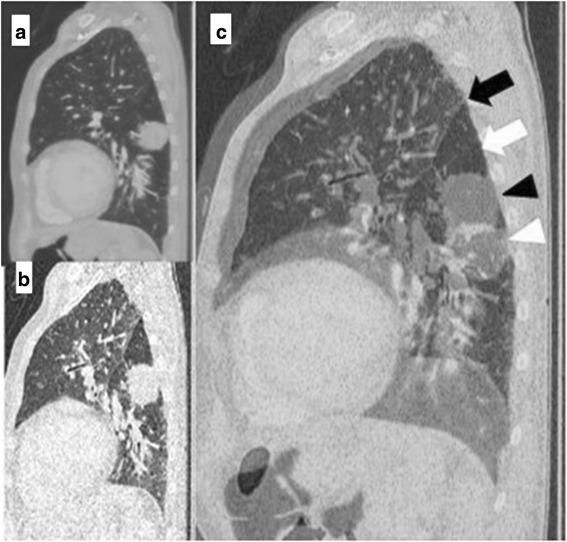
Images of a case of no pleural adhesion (adhesion grade 0). a Sagittal CT image of inspiration phase. b Sagittal CT image of expiration phase. c Two images superimposed. Significant fissure lines and tumor movements were seen. White arrow indicates fissure line in inspiration phase. White arrow head indicates a tumor in inspiration phase. Black arrow indicates fissure line in expiration phase. Black arrow head indicates a tumor in expiration phase
